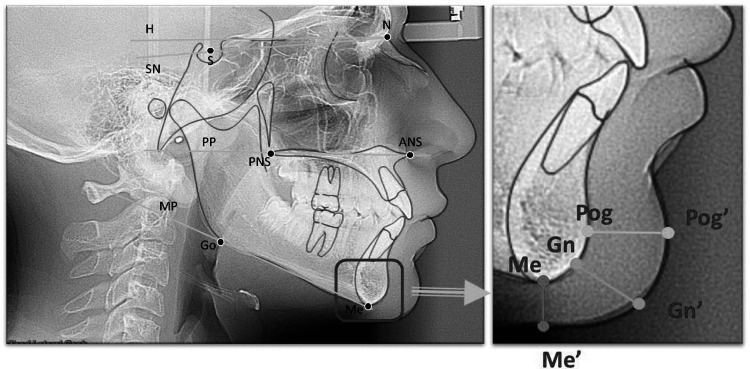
Radiographic anatomic landmarks of the mandible and chin used for measurement To the left: Mandibular plane (menton-gonion) and sella-nasion plane angle. To the right: Measurements of skin thickness at the chin were evaluated: hard tissue pogonion and the distance to its horizontal projection on the soft tissue (Pog-Pog’), distance from hard tissue gnathion to soft tissue gnathion (Gn-Gn’), and the distance from hard tissue menton to its vertical projection on the soft tissue (Me-Me’) Source: Macari AT, Hanna AE. Comparisons of soft tissue chin thickness in adult patients with various mandibular divergence patterns. The Angle Orthodontist. 2014. [11] (Open Access)
